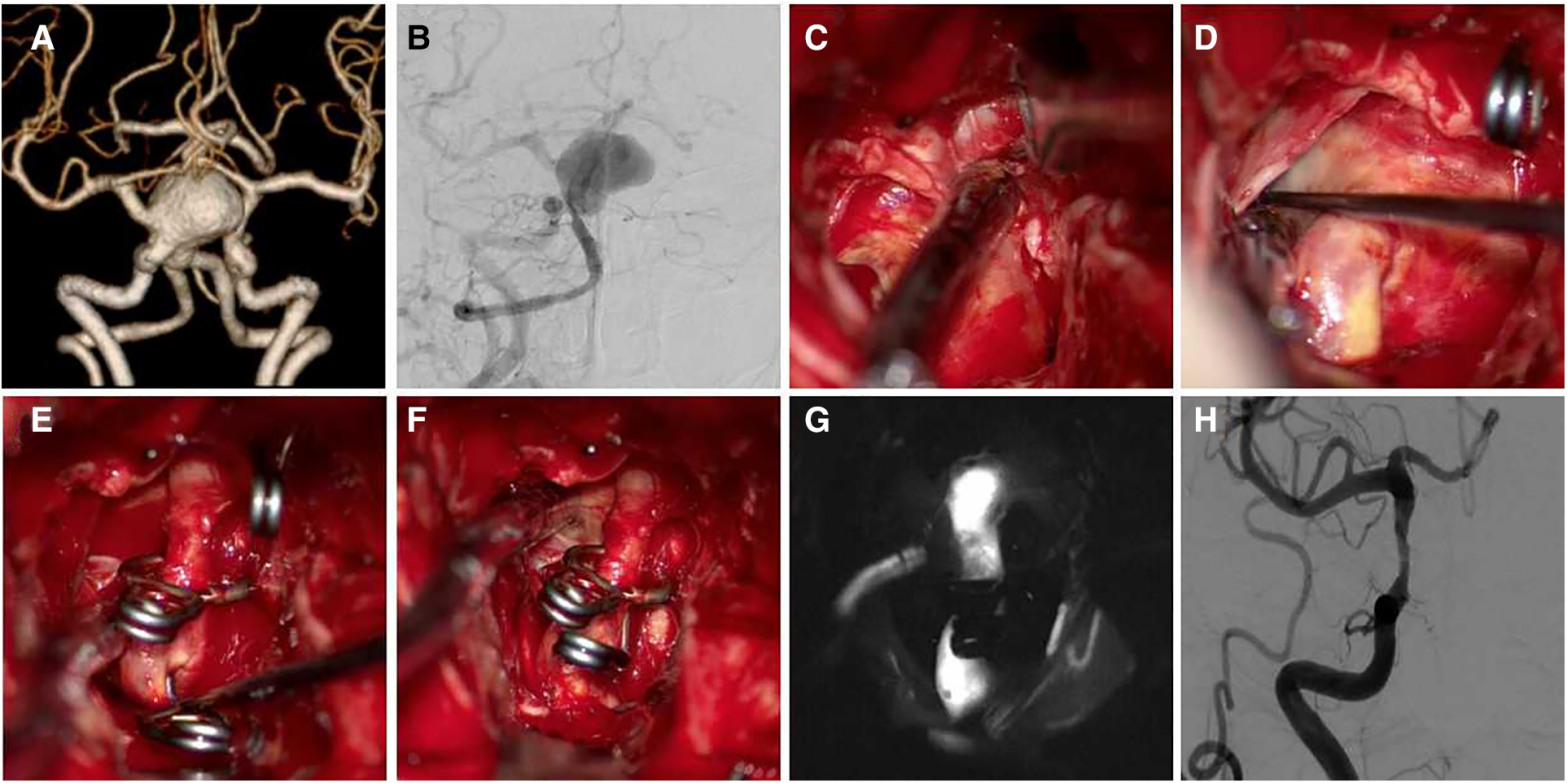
A 61-year-old patient presented with blurred vision in the right eye, which had lasted 6 months. (A,B) Preoperative CTA or DSA showed an ophthalmic aneurysm located on the ventral-medial wall of the parent artery; (C) exposure and temporary occlusion of the clinoid segment of the ICA; (D) the parent artery is dilated, and it is difficult to distinguish the neck of the aneurysm; (E) “T” shaped clip was used to clip the aneurysm, and the aneurysm residual at the distal end was seen; (F) A fenestrated-angled clip was used to clip the residual; (G,H) ICG in surgery, DSA follow-up showed patency and mild stenosis of parent artery.
